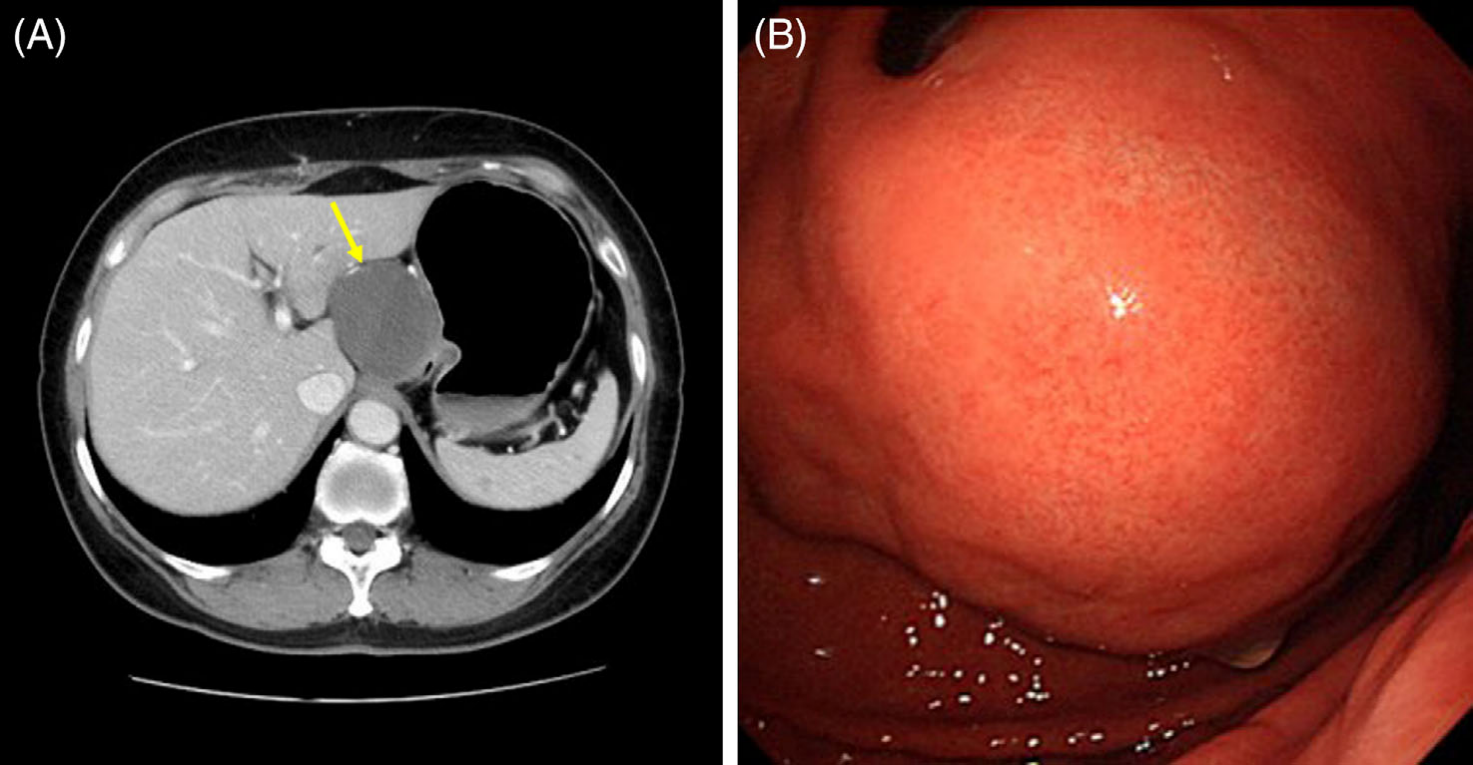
A, Esophagogastroduodenoscopic and B, abdominal CT images of an abdominal esophageal submucosal tumor. A 70‐mm submucosal tumor without mucosal defects in the abdominal esophagus was observed. The yellow arrow indicates the submucosal tumor in the abdominal esophagus
